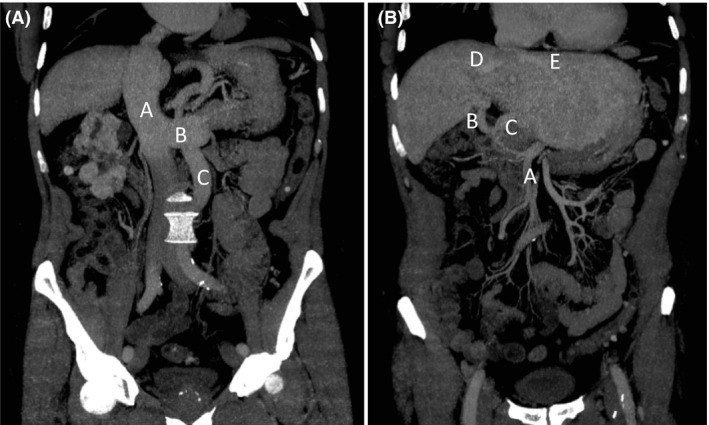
The coronal section of the CT scan showed (A) a type 1a Abernethy malformation, with complete agenesis of the portal vein, and (B) the arterialisation of the liver before embolization and the absence of the middle hepatic vein. (A) A: vena cava; B: left renal vein; C: inferior mesenteric vein. (B) A: superior mesenteric artery; B: right hepatic artery; C: left hepatic artery; D: right hepatic vein; E: left hepatic vein.
